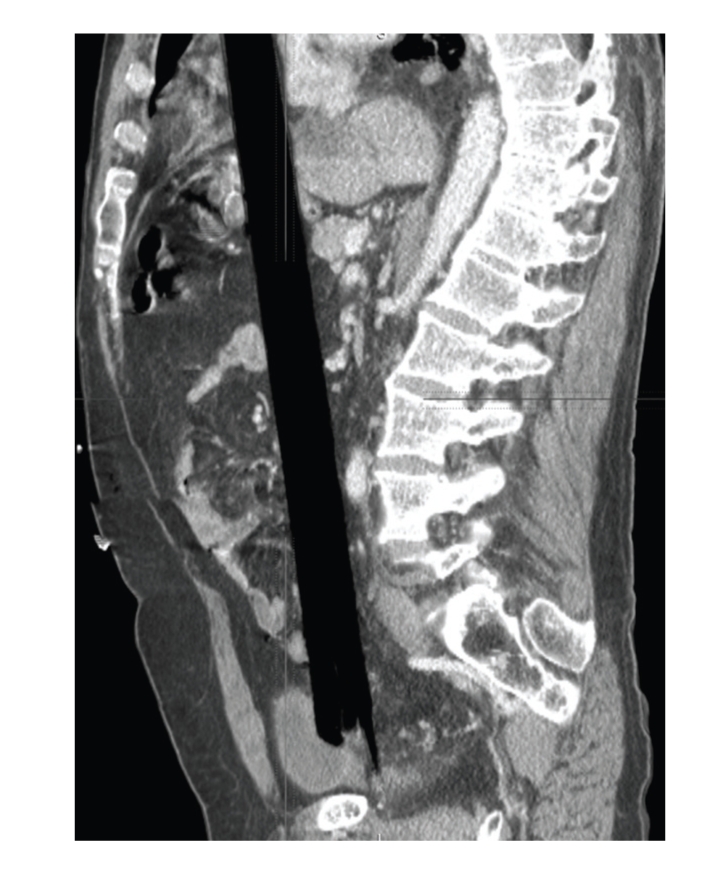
Sagittal CT radiograph showing the splintered end of the impaled object.
